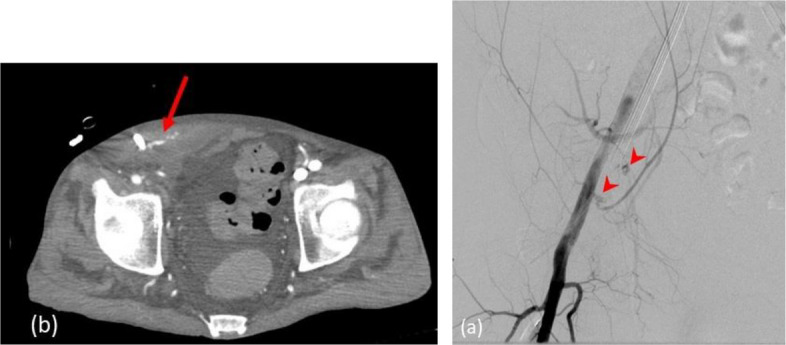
Hematoma in a 45-year-old man. A 12-Fr temporary hemodialysis catheter was placed in the right femoral vein for management of chronic kidney disease, after which hematoma developed in the groin and anterior abdominal wall. a Contrast-enhanced computed tomography suggested injury to the right inferior epigastric artery (arrow). b Angiography confirmed injury to the right inferior epigastric artery with active extravasation from an inferior epigastric artery branch (arrowheads). Transcatheter arterial embolization was performed with N-butyl-2-cyanoacrylate
